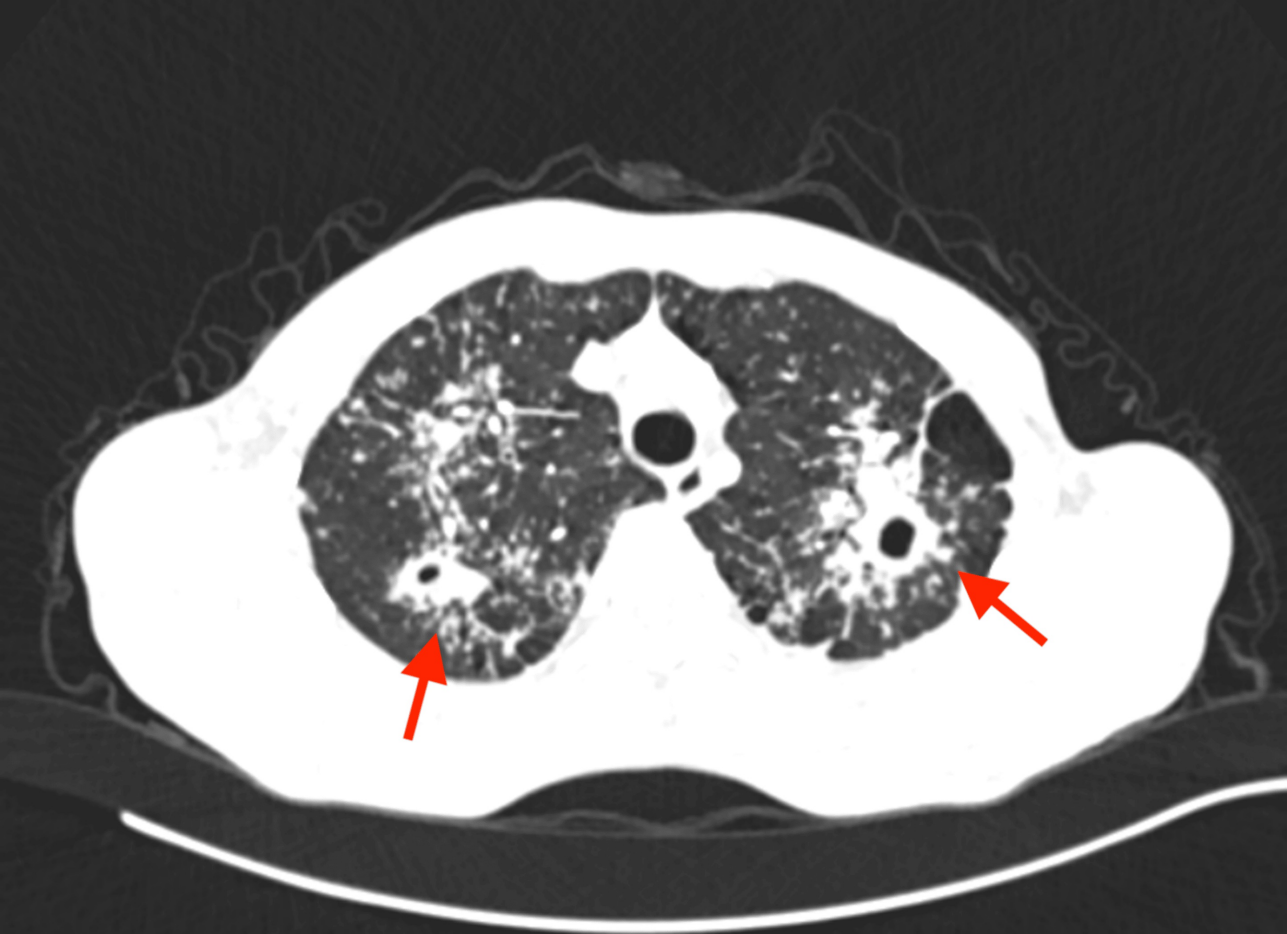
Chest CT scan showing caverns in both upper lobes (arrows).
